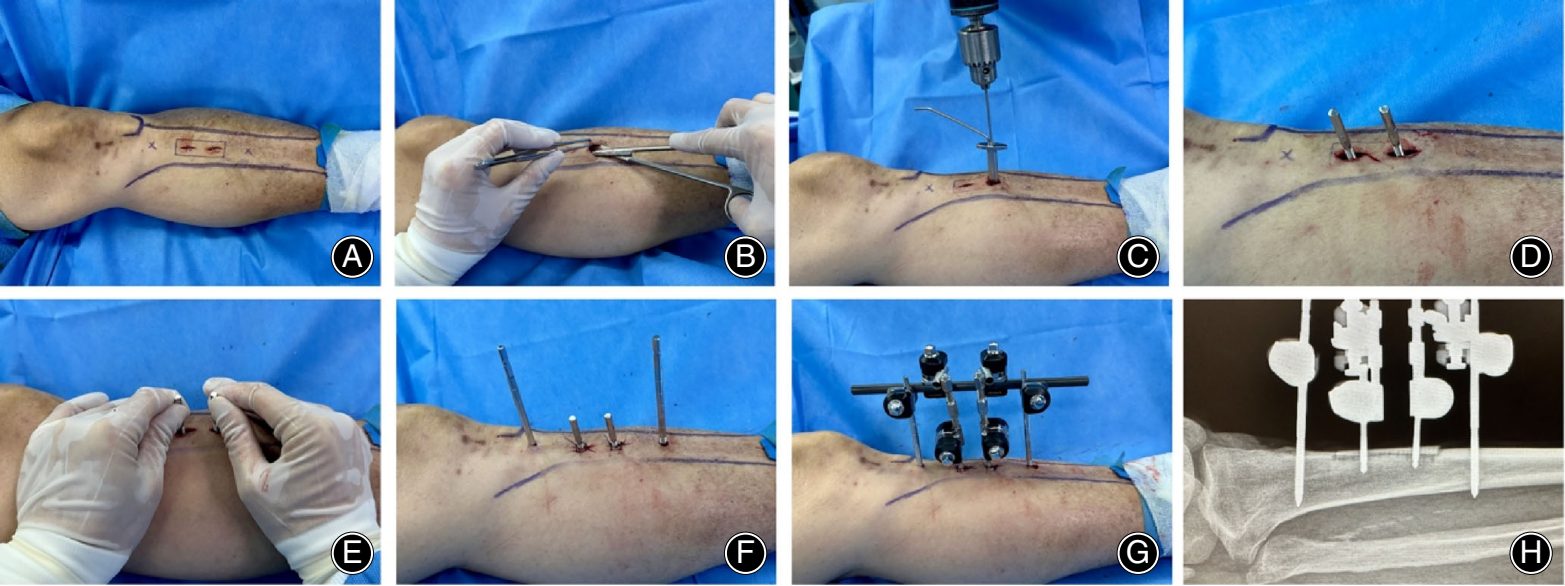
Surgical procedure for Tibial cortex transverse transport (TTT). (A) Preoperative marking of the surgical area and creation of two longitudinal skin incisions. (B) Dissection and exposure of the subcutaneous tissue over the periosteal surface. (C) Execution of successive perforations to facilitate pre‐truncation. (D) Insertion and securing of the transfer pin. (E) Isolation and removal of the bone block. (F, G) Insertion of fixation pins and assembly of the external fixation and transfer apparatus. (H) Postoperative radiographic confirmation of successful corticotomy interception.
